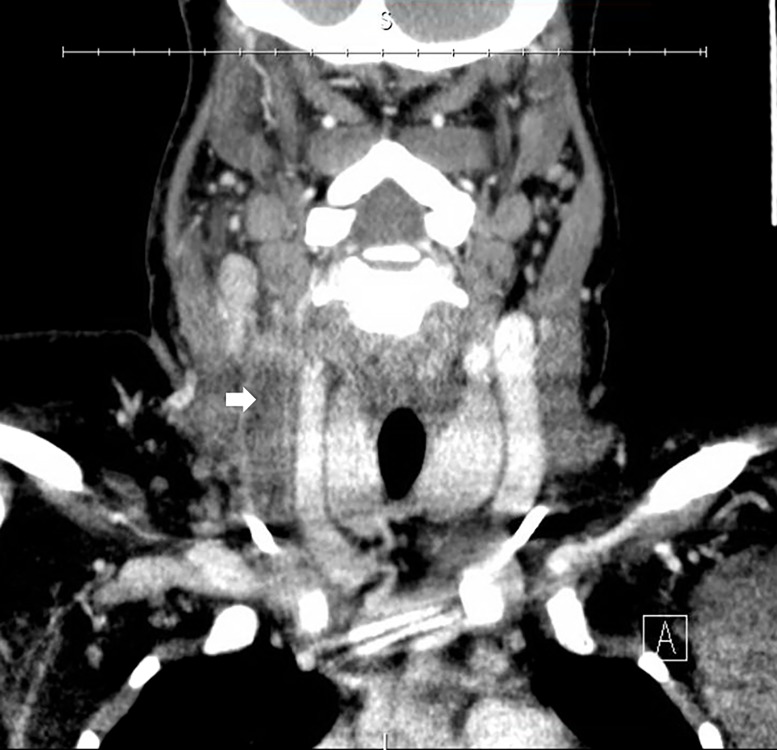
Coronal view of a computed tomography of the neck with contrast. Arrow indicates thrombus in the right internal jugular vein with complete occlusion.
